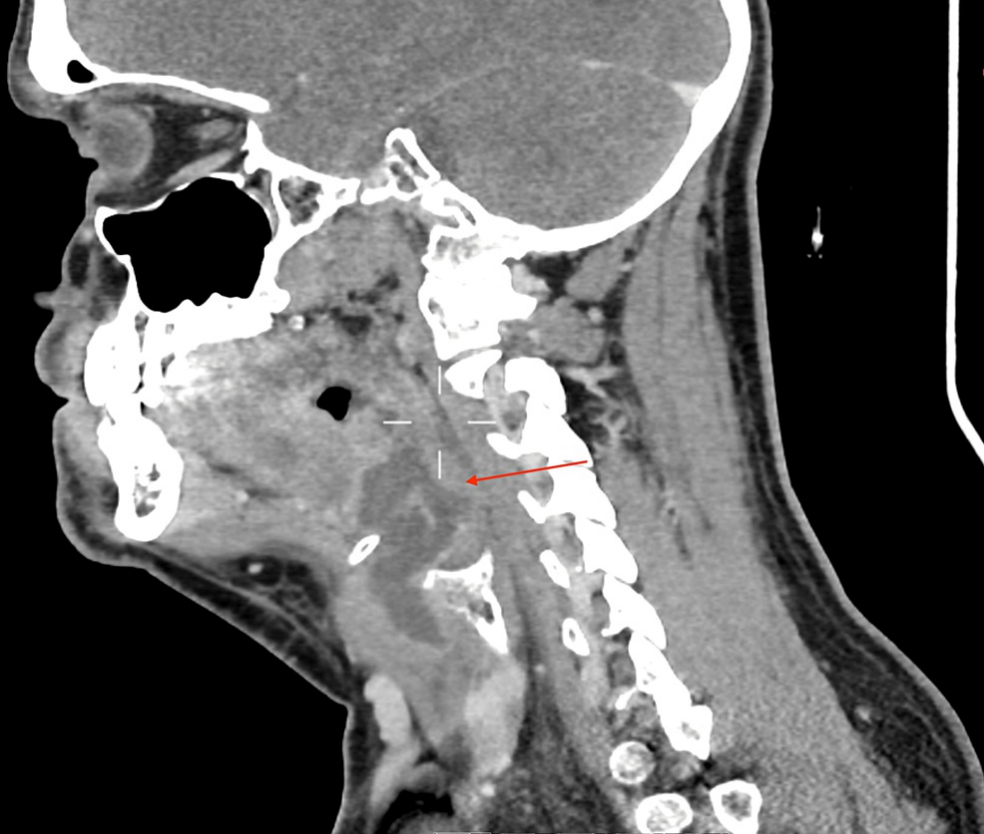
Sagittal view of a CT neck demonstrating a left-sided parapharyngeal collection (red arrow)
